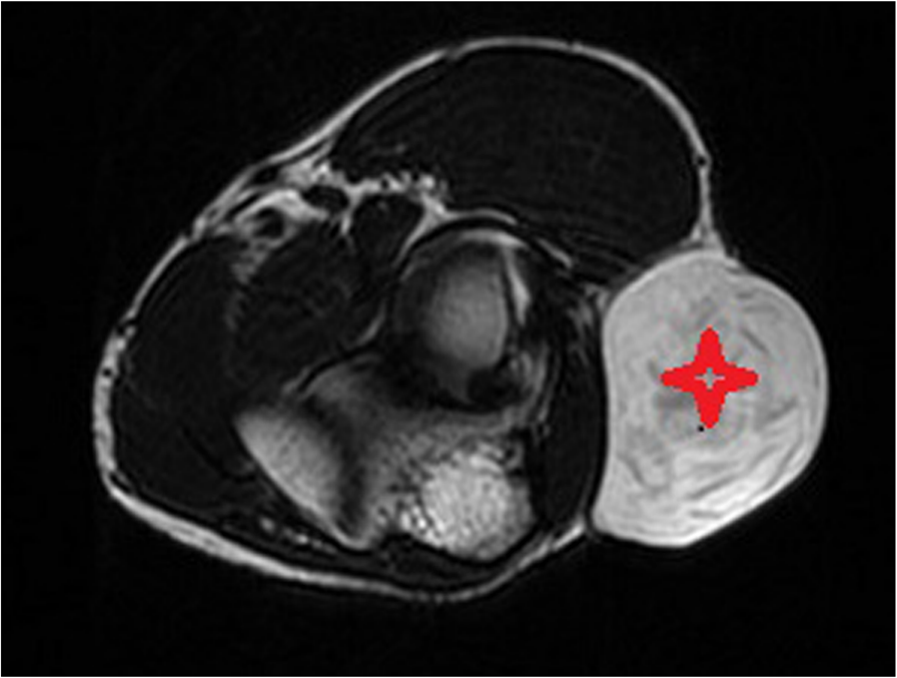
MRI image; T2 axial view showing unilocular cyst with thin septations
